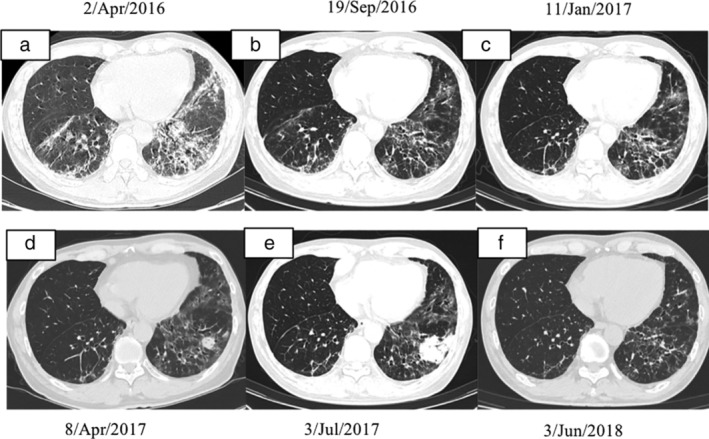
Serial high‐resolution CT scans of the chest. (a) The CT scan on 2 April 2016 showed reticular opacities, irregular linear opacities and diffuse ground‐glass opacities in both inferior lungs and left lingual lobe. (b) The CT scan on 19 September 2016 showed that ground glass opacities in both inferior lungs and left lingual lobe was improved after treatment. (c) The CT scan on 11 January 2017 showed that ground glass opacities in both inferior lungs and left lingual lobe was stable during follow‐up. (d) The CT scan on 8 April 2017 showed a new onset nodule in the left inferior lung, and the interstitial lung disease was stable. (e) The CT scan on 3 July 2017 showed the pulmonary nodule in the left inferior lung was obviously enlarged, but the ground glass opacities were stable. (f) The CT scan on 3 June 2018 showed that the left inferior pulmonary nodule had disappeared, and the ground‐glass opacities in both lungs had also improved.
